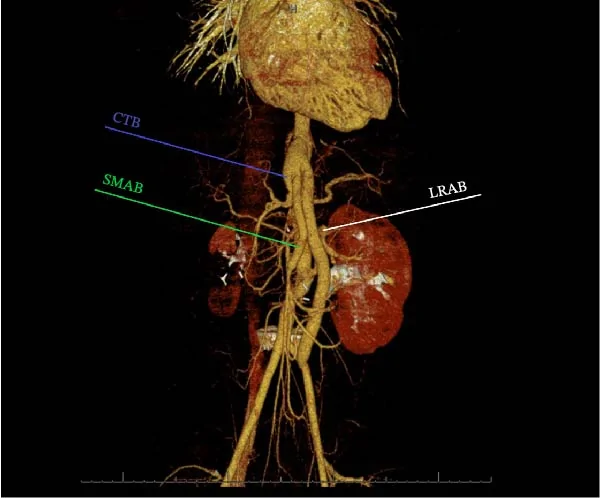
3D‐reconstruction of the CT angiography of the definitive reconstruction at follow‐up after six months. The Y‐graft arises from the supraceliac aorta. Its limbs supply the celiac trunk (end‐to‐end, CTB) and the SMA (end‐to‐side onto the lateral wall of the more cranial SMA segment, SMAB). The 6 mm graft to the right renal artery originates from the iliac jump‐graft, which, in this CT is already occluded and thus not visible. The bypass for the left renal artery (LRAB) remained unchanged.
